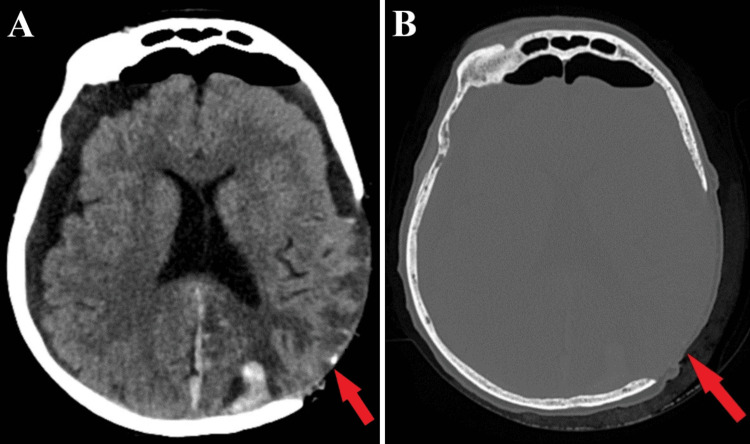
Postoperative CT scan aspect CT scan of tissue window (A) and bone window (B). In both images, the skull segment was not placed postoperatively (red arrows) due to significant compressive effects.
